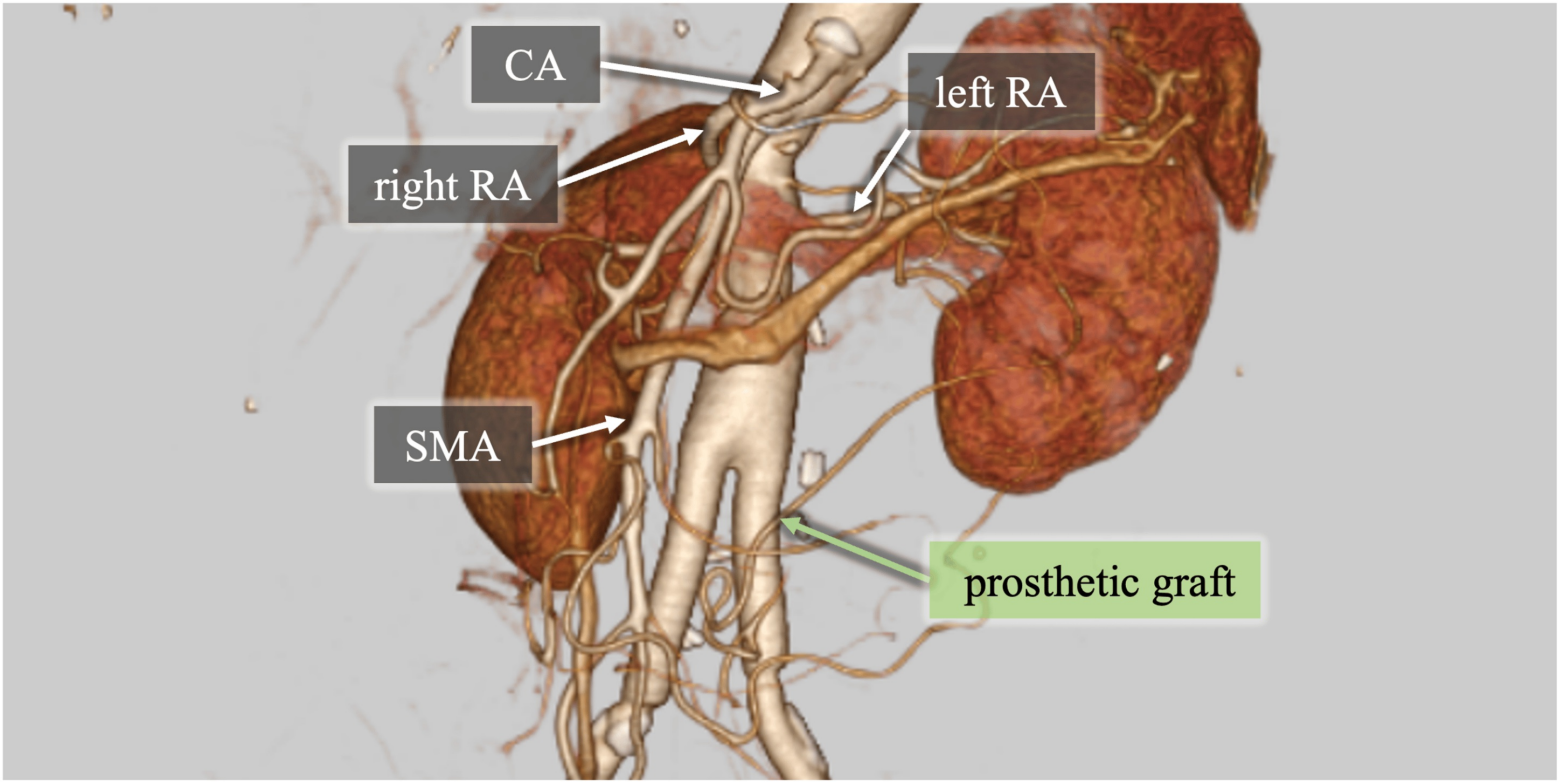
Postoperative CT imaging All anastomotic sites showed no significant stenosis, and the arteries to both lower limbs remained patent. The CA, SMA, and both right and left renal arteries were consistent with their preoperative condition.
